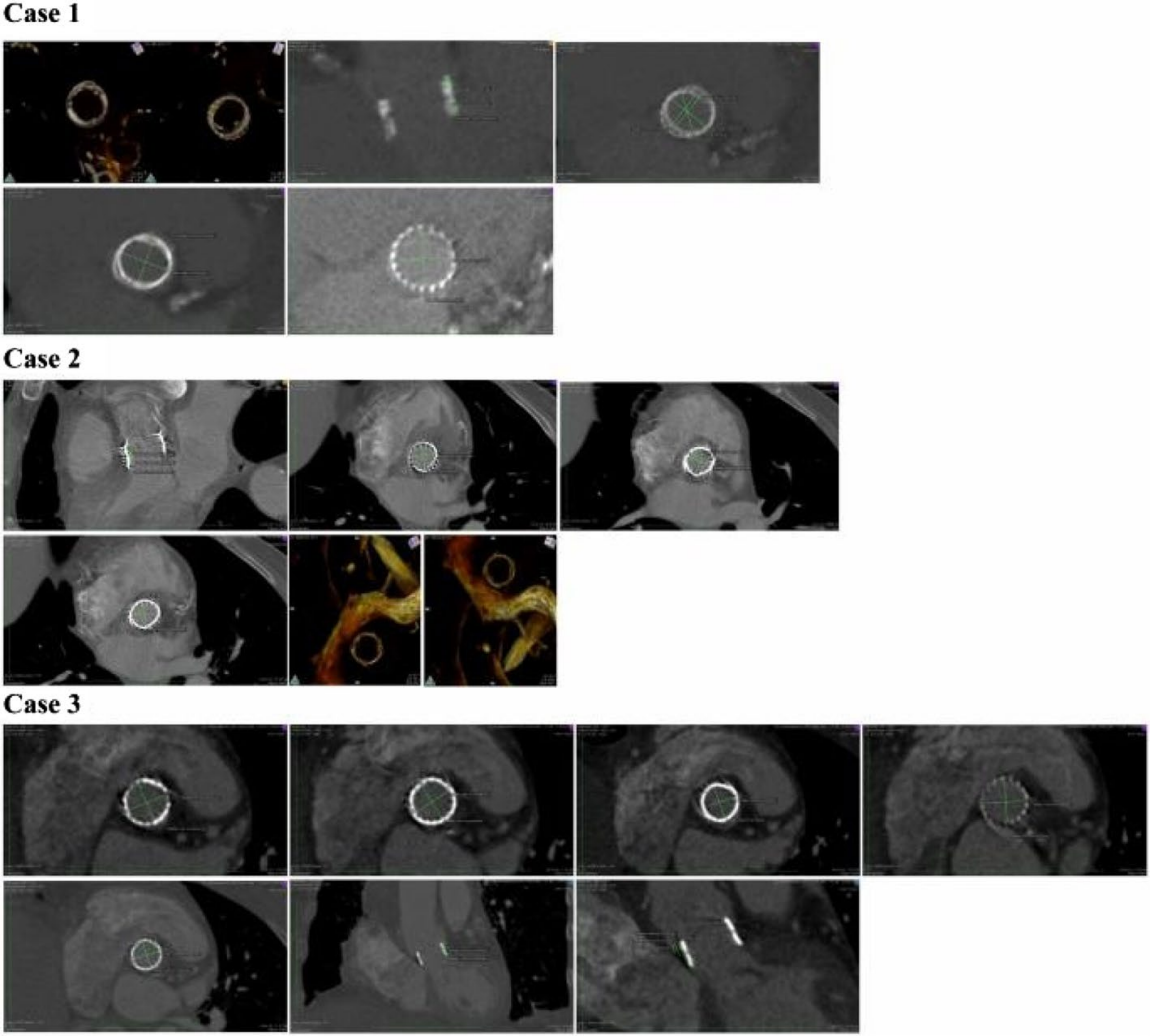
CT-Follow-up of Cases that received RDAVR with the Intuity image series obtained with OsiriX ™ providing us a pseudo-realistic three-dimensional depiction of the human body, highlighting the aortic bioprosthesis in situ with different views at different levels of the valve level, with the actual measurements taken on an appropriately reformatted CT scan
